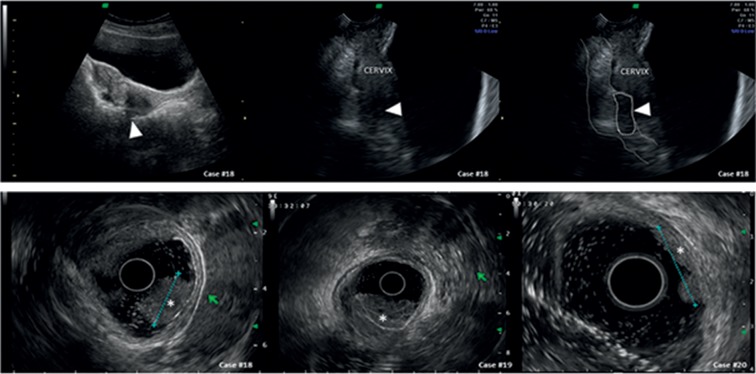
Differential diagnosis with bowel polyps and cancer (sonographic details of each case are reported in Table 4).
